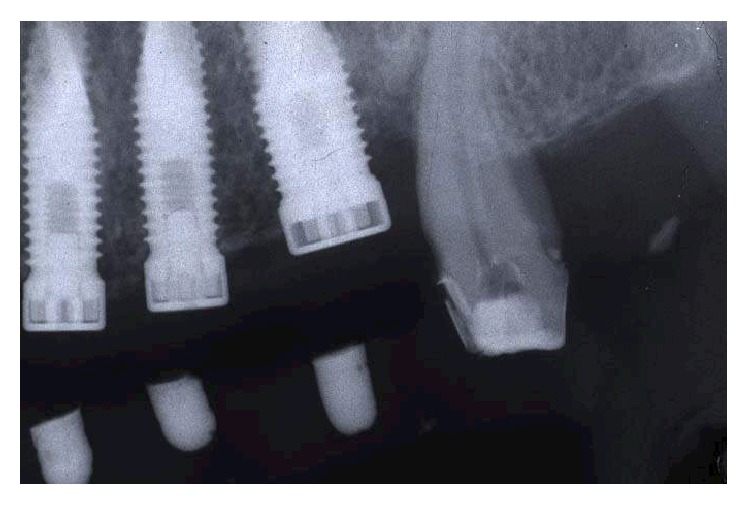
Radiographic postoperative control: coincidence of radiopaque landmarks embedded in the temporary crowns and the implant position of 24, 25, and 26.
